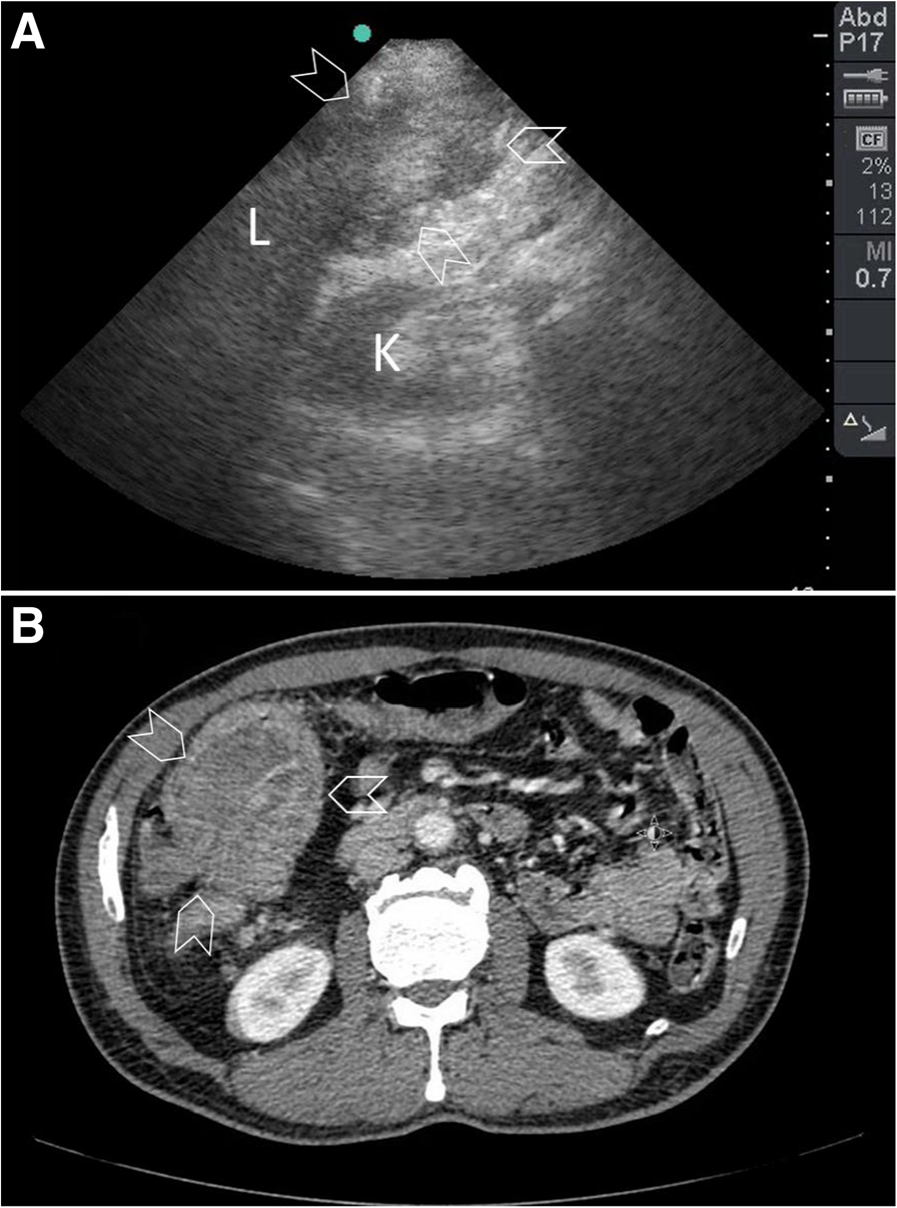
A 57-year-old man presented with abdominal pain and diarrhoea of 10 days duration. On abdominal examination, there was a mobile mass in the right upper quadrant. The abdomen was soft and not distended. The patient was anemic. POCUS study (a) using a portable ultrasound machine and a small print convex array probe having a frequency of 3–5 MHz has shown an irregular mass in the right colon without a lumen just under the liver and 10 cm long (arrowheads). A clinical diagnosis of right colonic malignancy was suspected. CT scan with intravenous contrast (b) has shown the same findings (arrowheads). The patient had a right hemicolectomy. The tumour was confirmed to be a poorly differentiated colonic adenocarcinoma. L liver, K kidney
